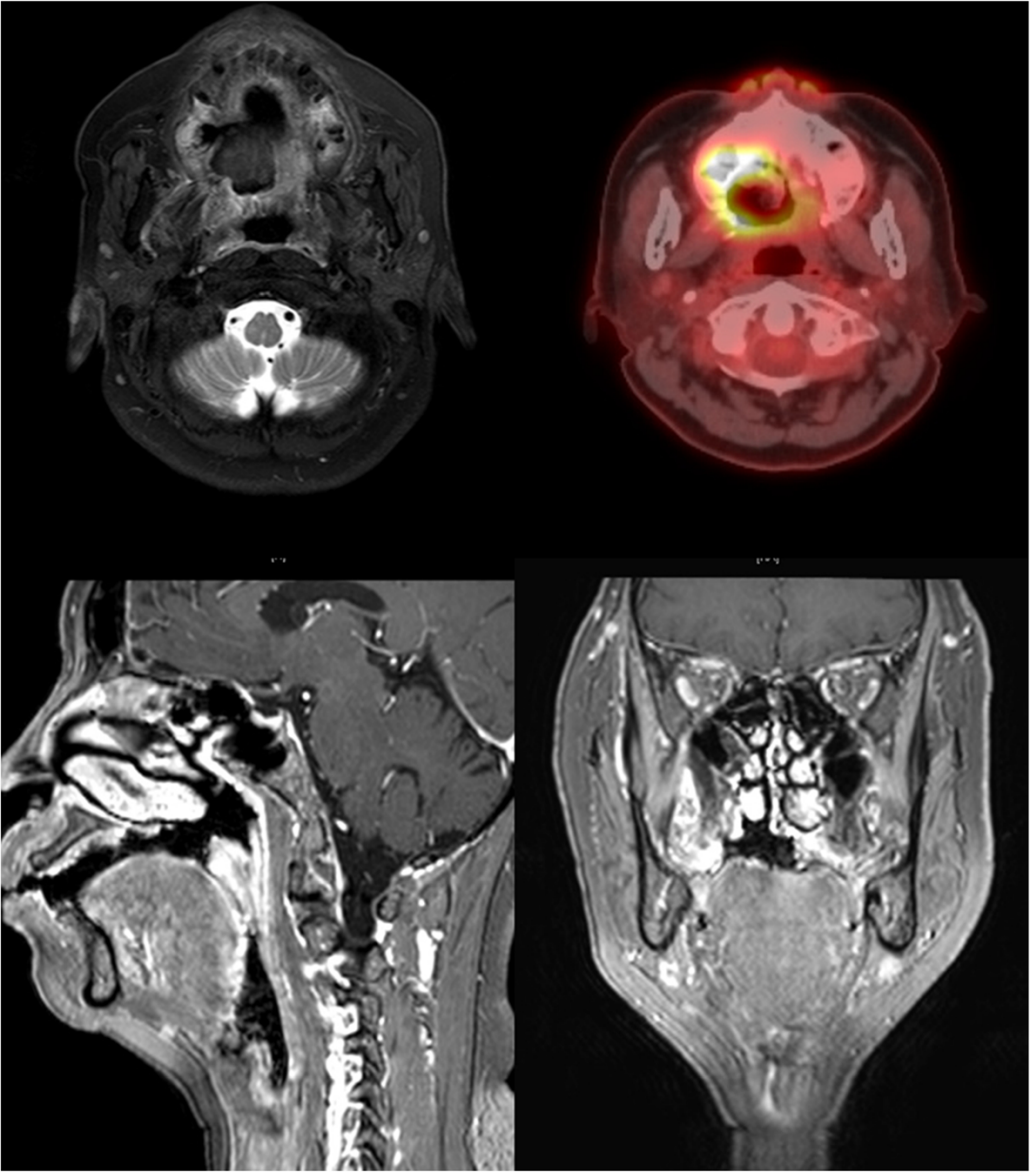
Magnetic resonance imaging (*top left* and *bottom*) and whole-body positron emission tomography (*top right*) showed no unusual finding following operation and mildly increased FDG uptake on the right hard palate, likely postoperative changes. There was no evidence of distant metastasis
